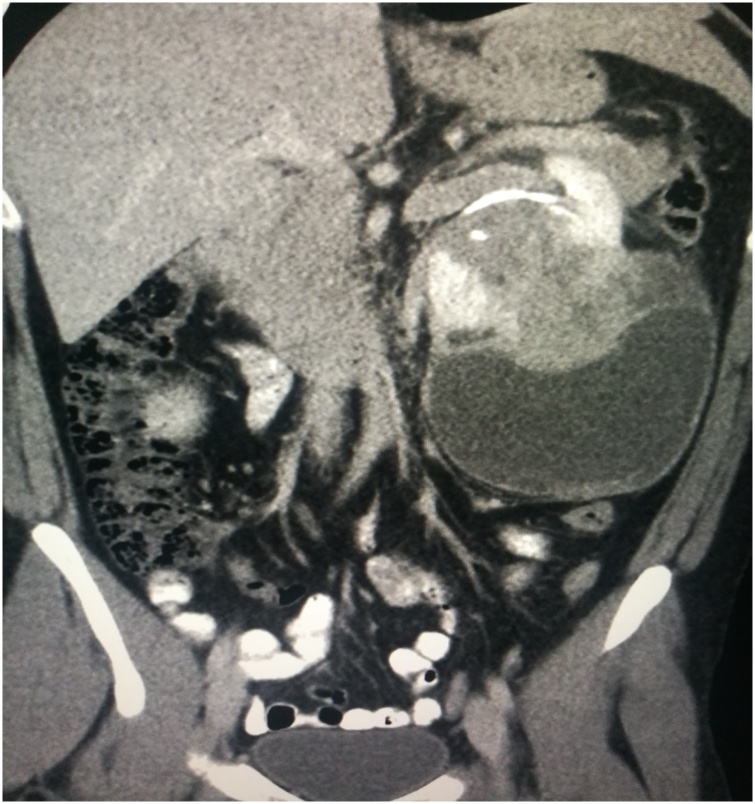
CT scan-Coronal view demonstrating huge left renal mass.
